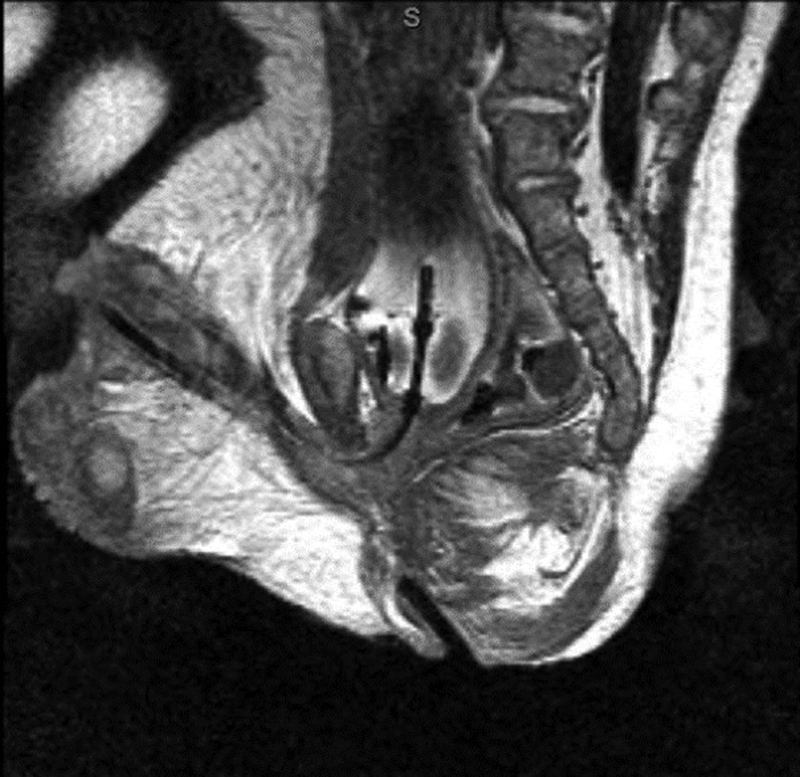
Sagittal magnetic resonance image (MRI) showing the superficial sphincter and deep sphincter complex, as well as the levator muscles. Note a Foley catheter balloon within the bladder as well as an MR-compatible needle within the first 1.5 cm of the sphincter complex.
